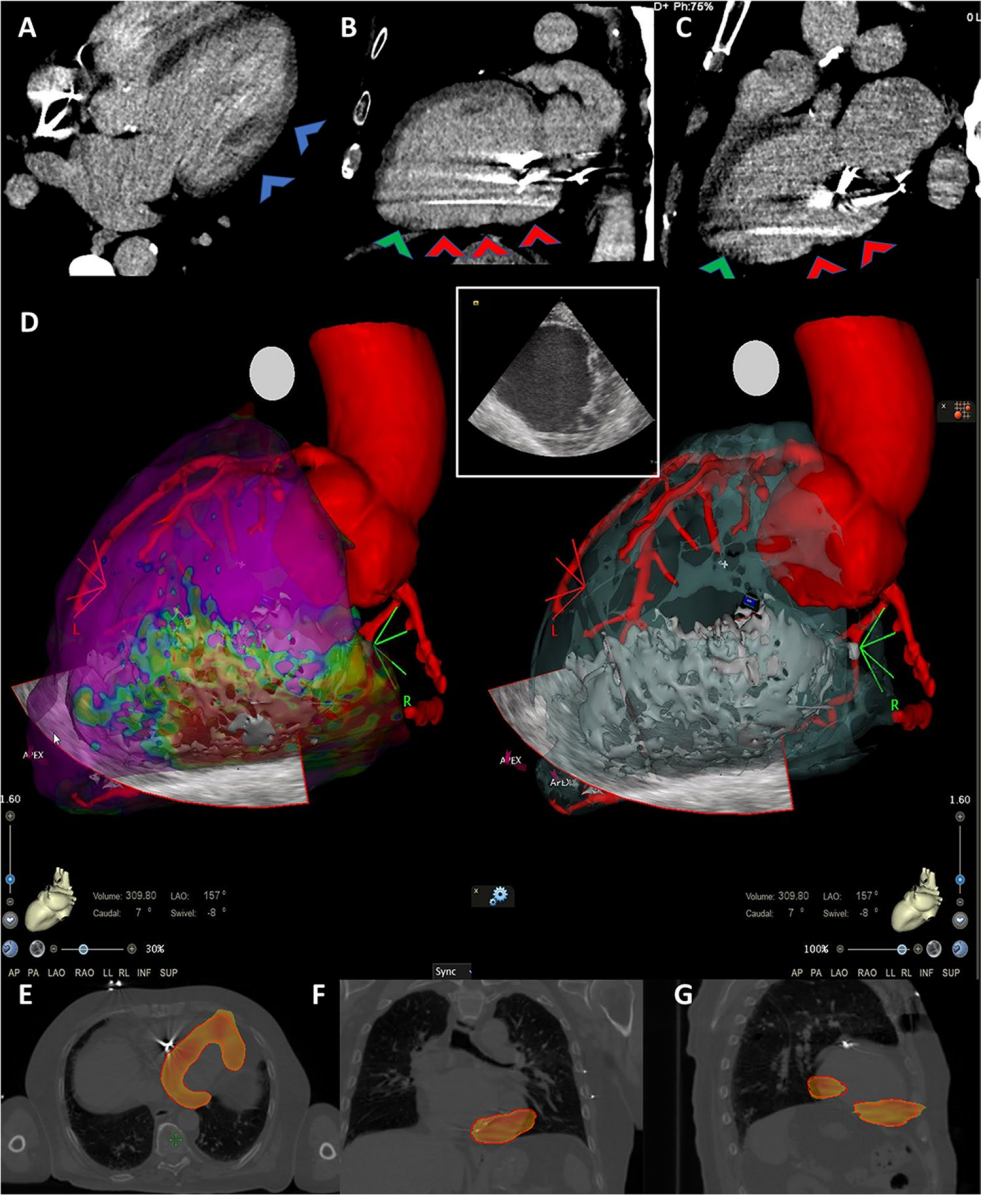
CT imaging with myocardial fibrosis evaluation, integrated electroanatomical mapping, and SBRT-treatment plan in one patient undergoing SBRT are represented. *Upper panels.* CT myocardial fibrosis evaluation in the long-axis view is represented. CT scan was acquired 8 min after the injection of iodinated contrast medium, as per protocol indication. No hyperdense myocardial areas are evident with regard to the anterolateral wall (A, blue arrowheads). Areas of hyperdense myocardium are shown (B, C): red arrowheads point at a transmural (ischemic pattern) myocardial fibrosis in the inferior and posterolateral wall of the left ventricle. The left ventricular apex appears free from lesions (green arrowheads). *Middle panel.* High-density epicardial electroanatomical mapping combined with CT imaging (D). Mid- and basal segments of the inferior and posterior wall are covered by diseased electrograms, represented in red and yellow by the “color-coded” map, as expression of the underlying electrical scar (left). In the same location, CT shows a pattern of discrete transmural fibrosis, which perfectly matches with the lesion revealed by the electroanatomical map (right). The intracardiac echo fan acquired during the mapping procedure is visualized in the map with the corresponding view in the small box. *Lower panels.* The planning target volume in the axial (E), coronal (F), and sagittal (G) view, as delineated by the red contour line, is depicted. The colored area represents the dose coverage, from the 95% to the maximum of the prescription dose. *Abbreviation:* CT, computed tomography
